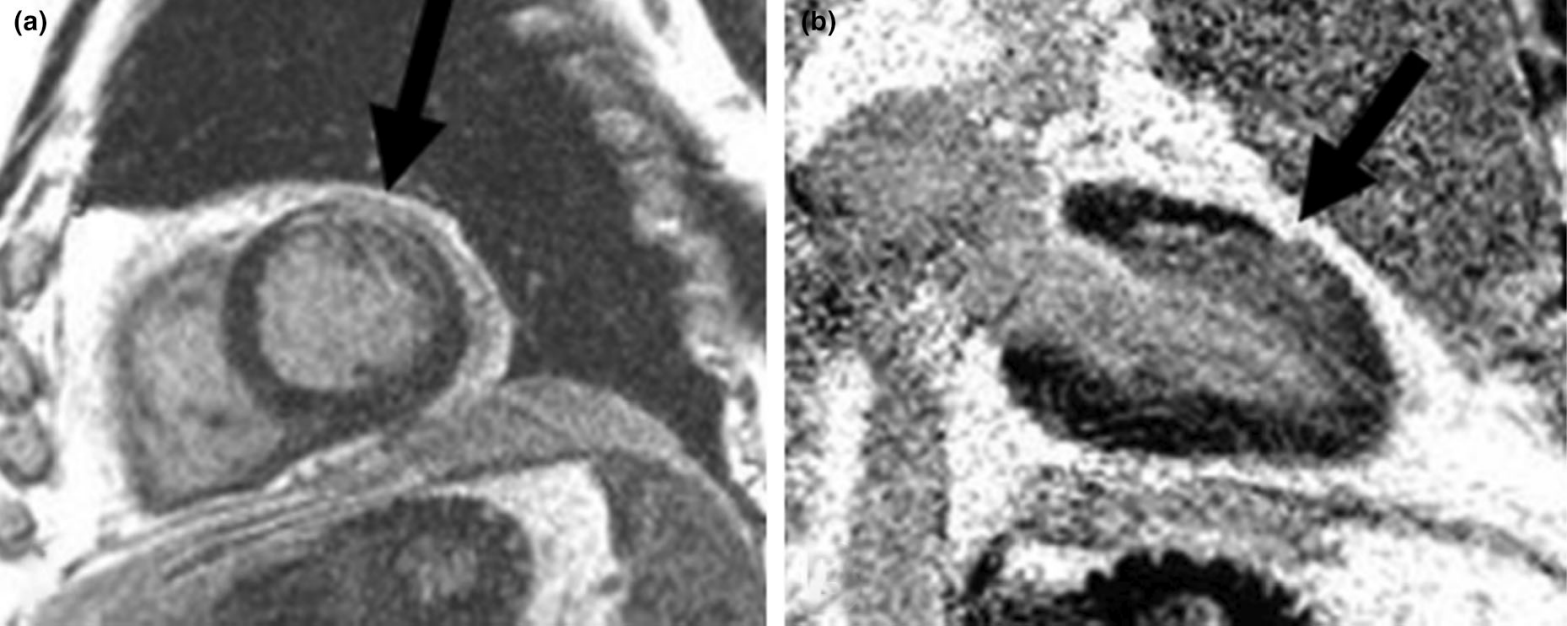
Sagittal (a) and coronal view (b) of a post gadolinium cardiac MRI of a 61‐year‐old male patient with GPA showing left ventricular wall myocardial late gadolinium enhancement in the anterior and anterolateral segments (arrows), indicative of myocarditis/fibrosis.
